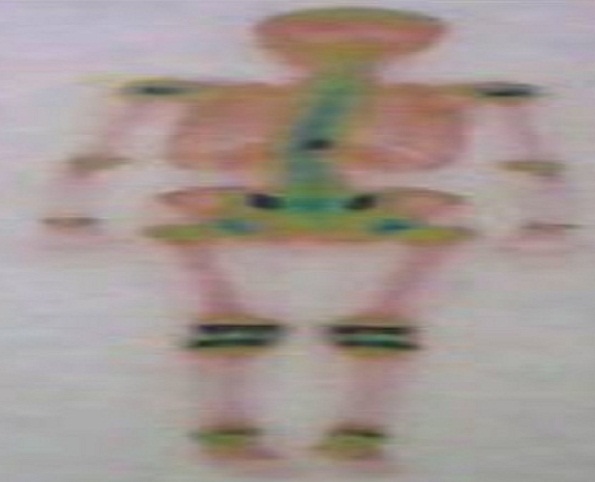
La scintigraphie osseuse (montre une volumineuse hyperfixation de la lame vertébrale droite de D11
